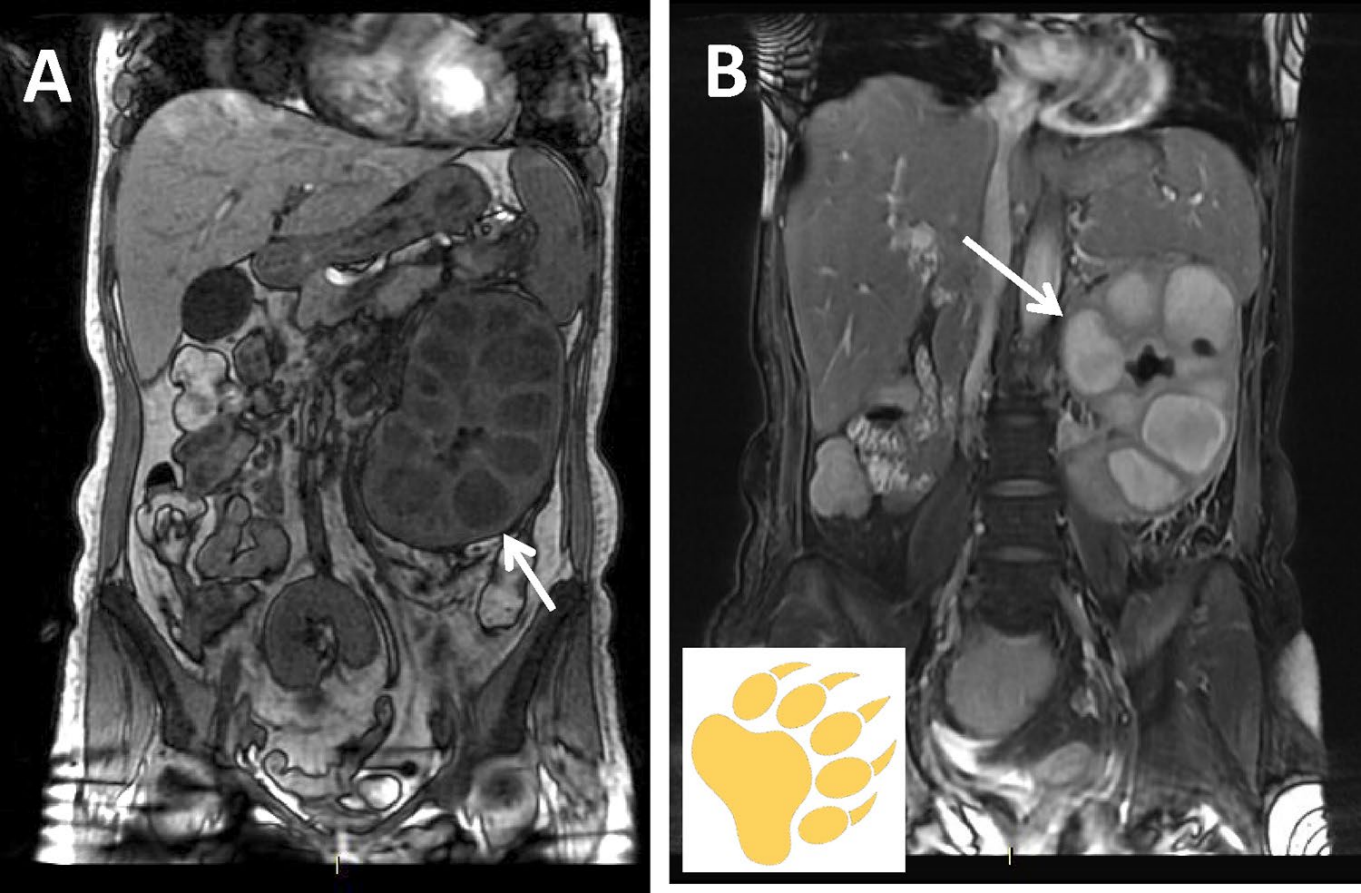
MRI appearance of xanthogranulomatous pyelonephritis. A 75-year-old female patient presenting with flank pain and fever. a Coronal T1-weighted images shows hypointense parenchymal cavities (arrow). b Coronal T2-weighted sequence shows hyperintense cavities (arrow) surrounded by a solid isointense rim and containing fluid levels, with upper more hyperintense component and lower more hypointense component, due to inflammatory debris and necrotic-purulent material; the combination of contracted renal pelvis, calyx dilatation and cortical thinning together constitutes the bear’s sign
